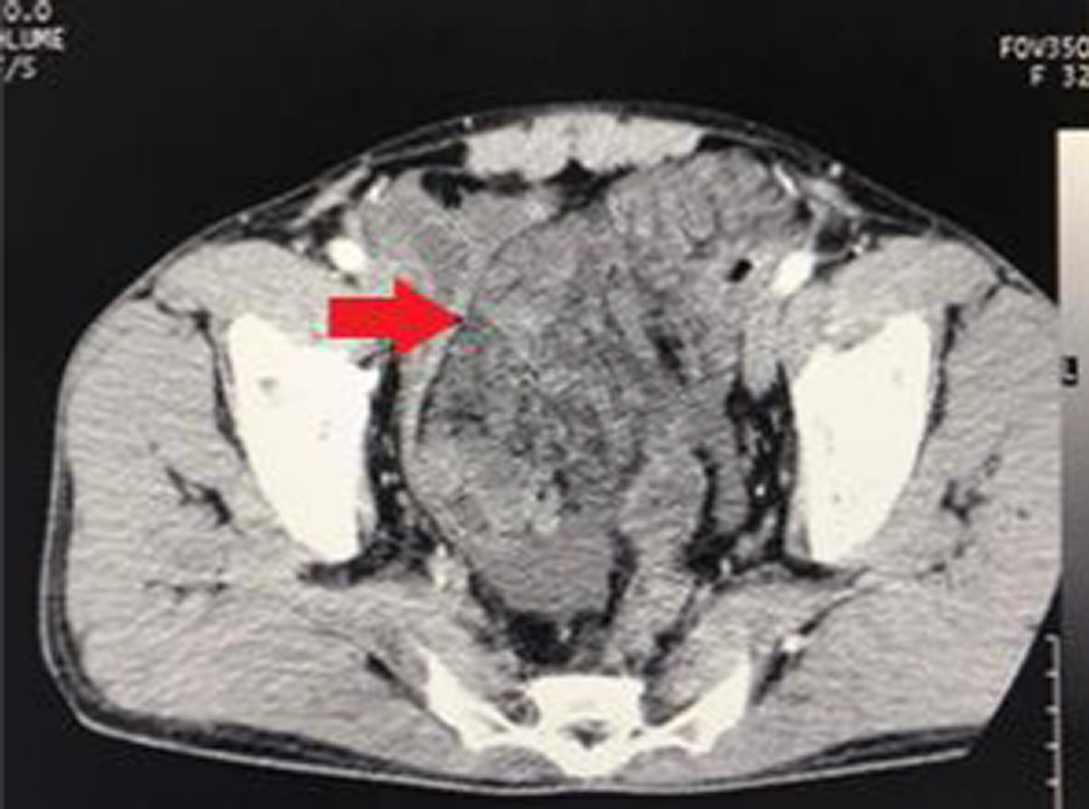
CT scan showing a huge mesenteric mass (arrow) in the pelvic region
